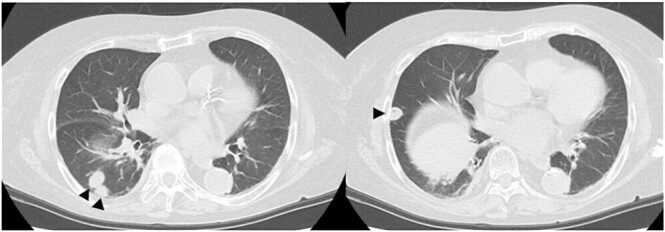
Non-contrast-enhanced computed tomography scan showed multiple nodular shadows in the right lung upon admission (black arrow heads).
